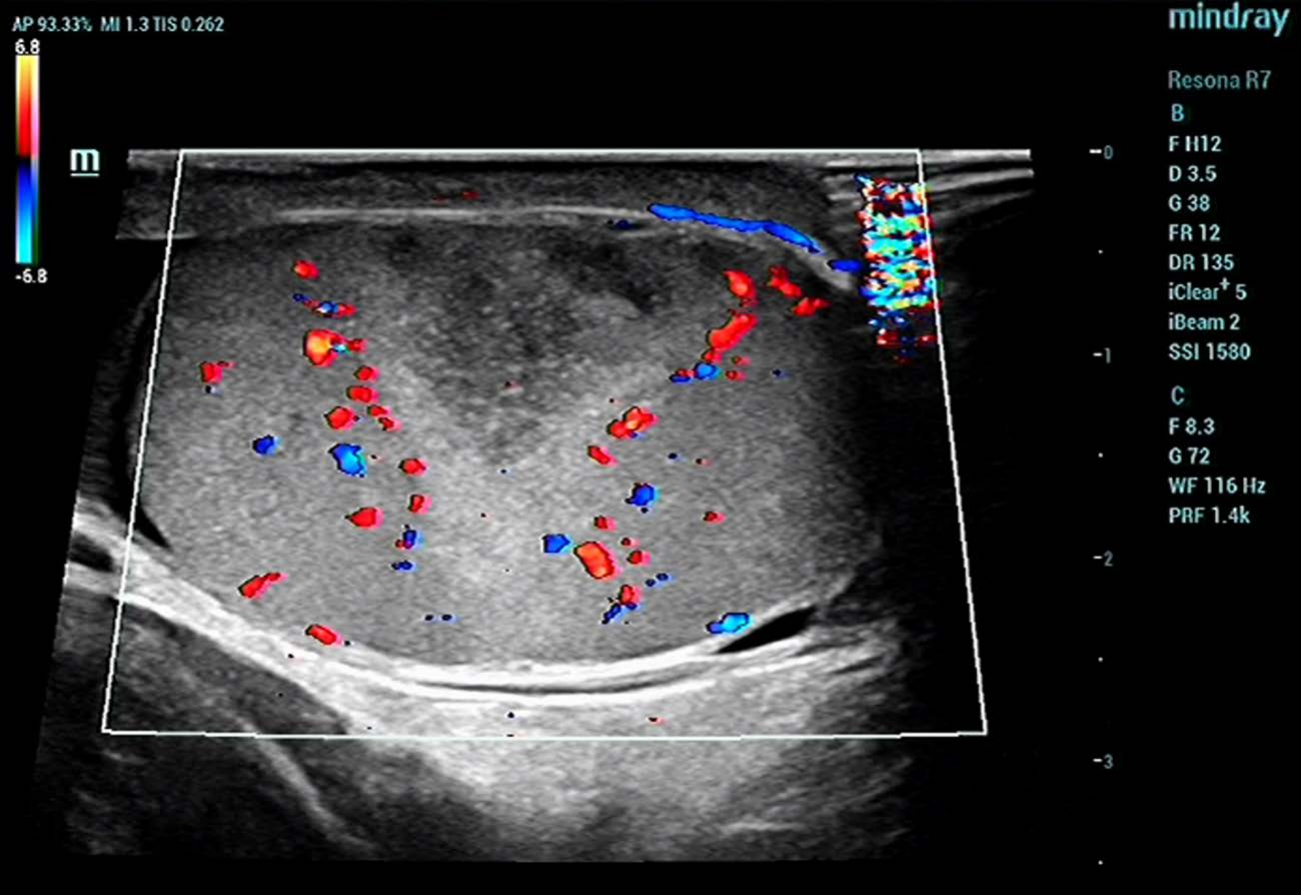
Color Doppler ultrasound of the testis that showed a hypoechoic area with an absent color flow in that area.
